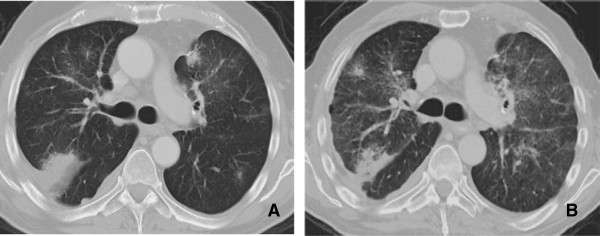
Interstitial Pneumonitis 58 year old male with NSCLC on bevacizumab. A: Pre treatment images demonstrate consolidative mass in the posterior segment of the right upper lobe. B: Post treatment CT shows marked reduction in size of a posterior segment RUL mass but with interval development of ground glass attenuation, interstitial thickening and parenchymal bands. Biopsy was performed and showed Interstitial pneumonitis.
